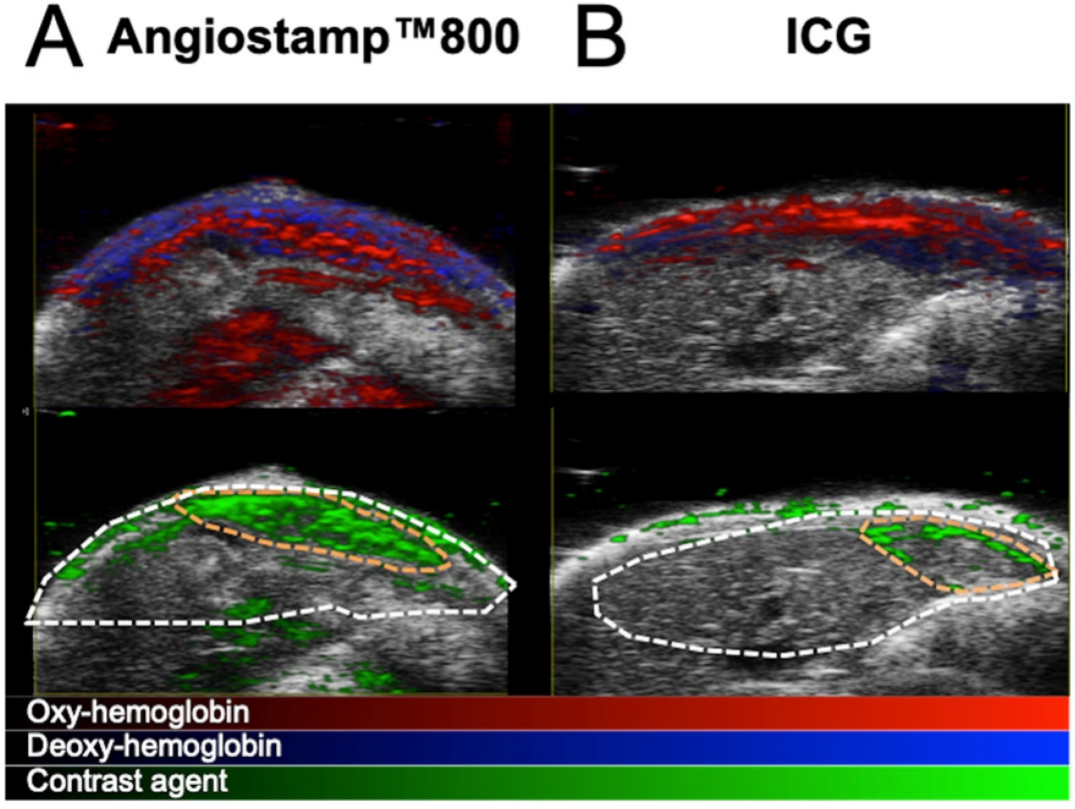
In vivo* mapping of oxyhemoglobin, deoxyhemoglobin and tracer distribution by multispectral photoacoustic imaging. Liver sections from 3D ultrasound and multispectral photoacoustic imaging with Angiostamp800 (A) or ICG (B) injected 24 hours or 1 hour before imaging, respectively (red: oxyhemoglobin, blue: deoxyhemoglobin, green: Angiostamp800 or ICG).
